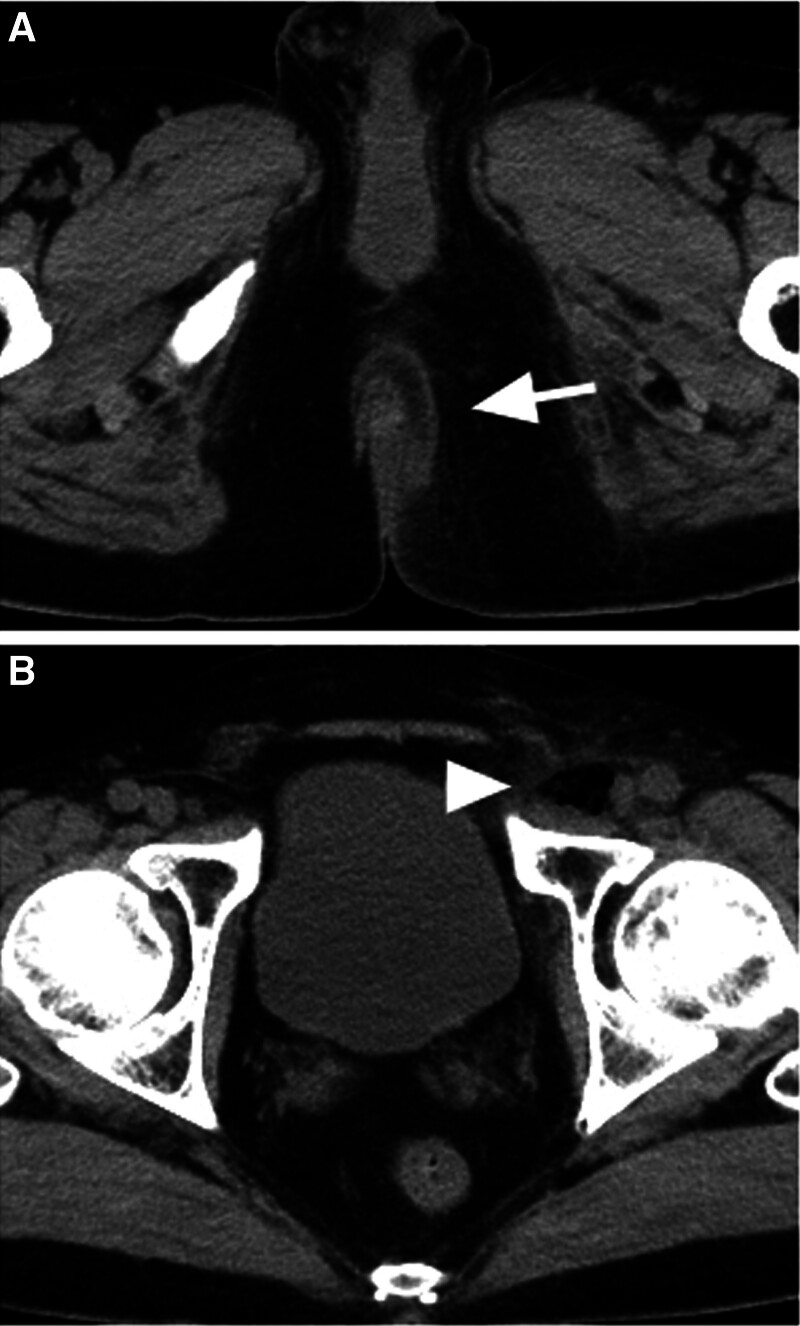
The computed tomography findings on the day of diagnosis. (A) The density of the soft tissue on the left side of the anus was elevated (white arrow). (B) Gas bubbles were found in the left femoral ring (white arrowhead).
